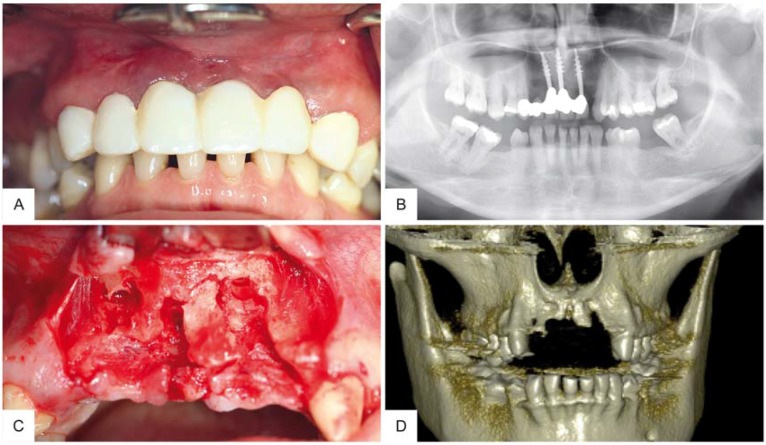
Clinical and radiographic examination of the maxillary defect. (A) Clinical preoperative examination revealed changed color in the gingiva on site #7–10; (B) Radiographic preoperative film demonstrated massive bone loss surrounding the three failing implants; (C) Complex bone defect and partial loss of the buccal wall within the maxillary aesthetic zone after extraction of failing implants. (D) CBCT image of the maxillary defect after implant extraction.
